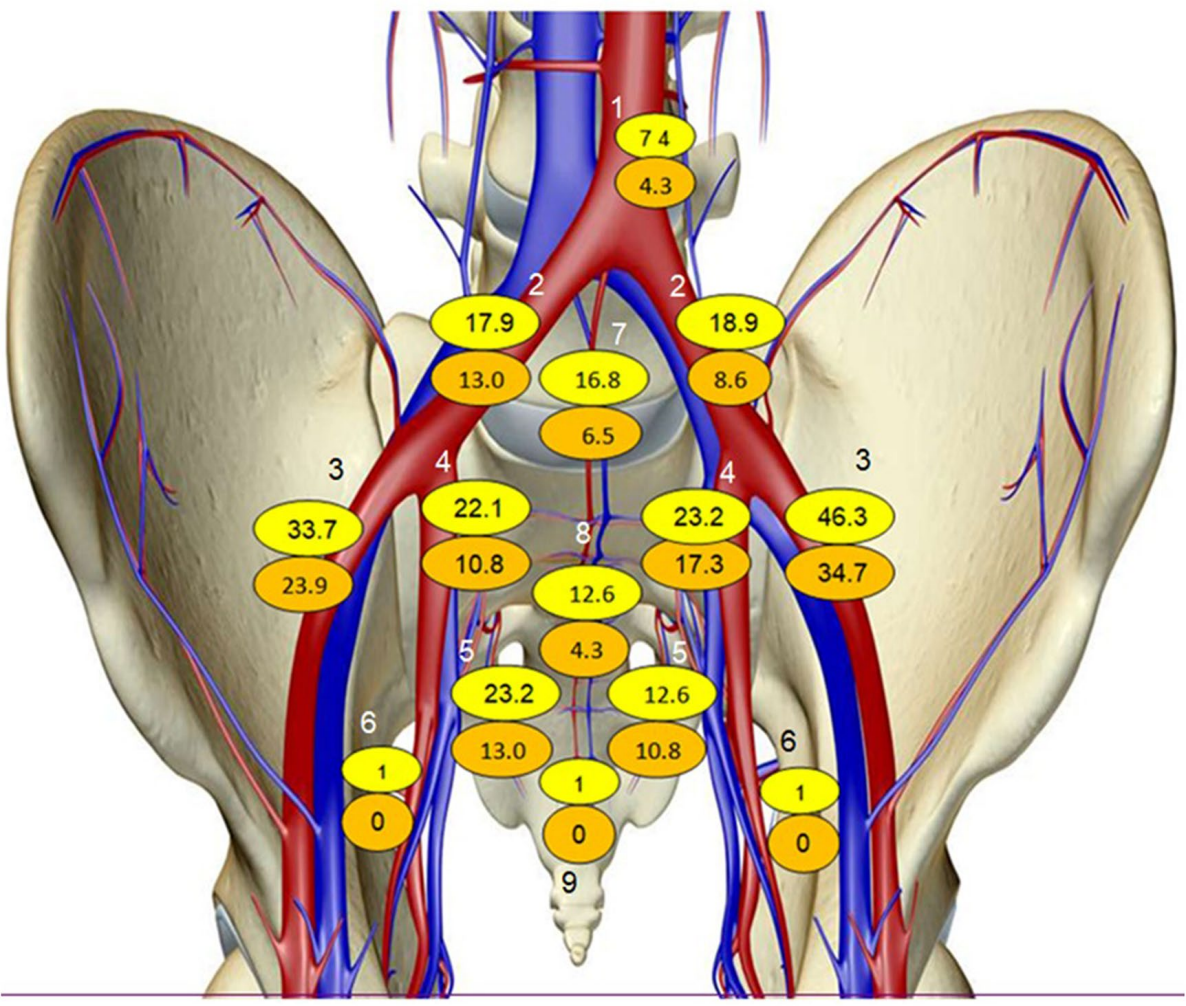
Lymph distribution (%) of all nodes (seen on both CT and PSMA PET) compared to PSMA PET only. Legend: Yellow = CT-positive/PSMA-positive nodes; Orange = CT-negative/PSMA-positive nodes 1-Aortic Bifurcation, 2-R + L Common Iliac. 3-R + L External Iliac. 4-R + L Internal Iliac. 5-R + L Obturator. 6-R + L Inguinal, 7-Presacral, 8-Mesorectal, 9-Prevesicle
